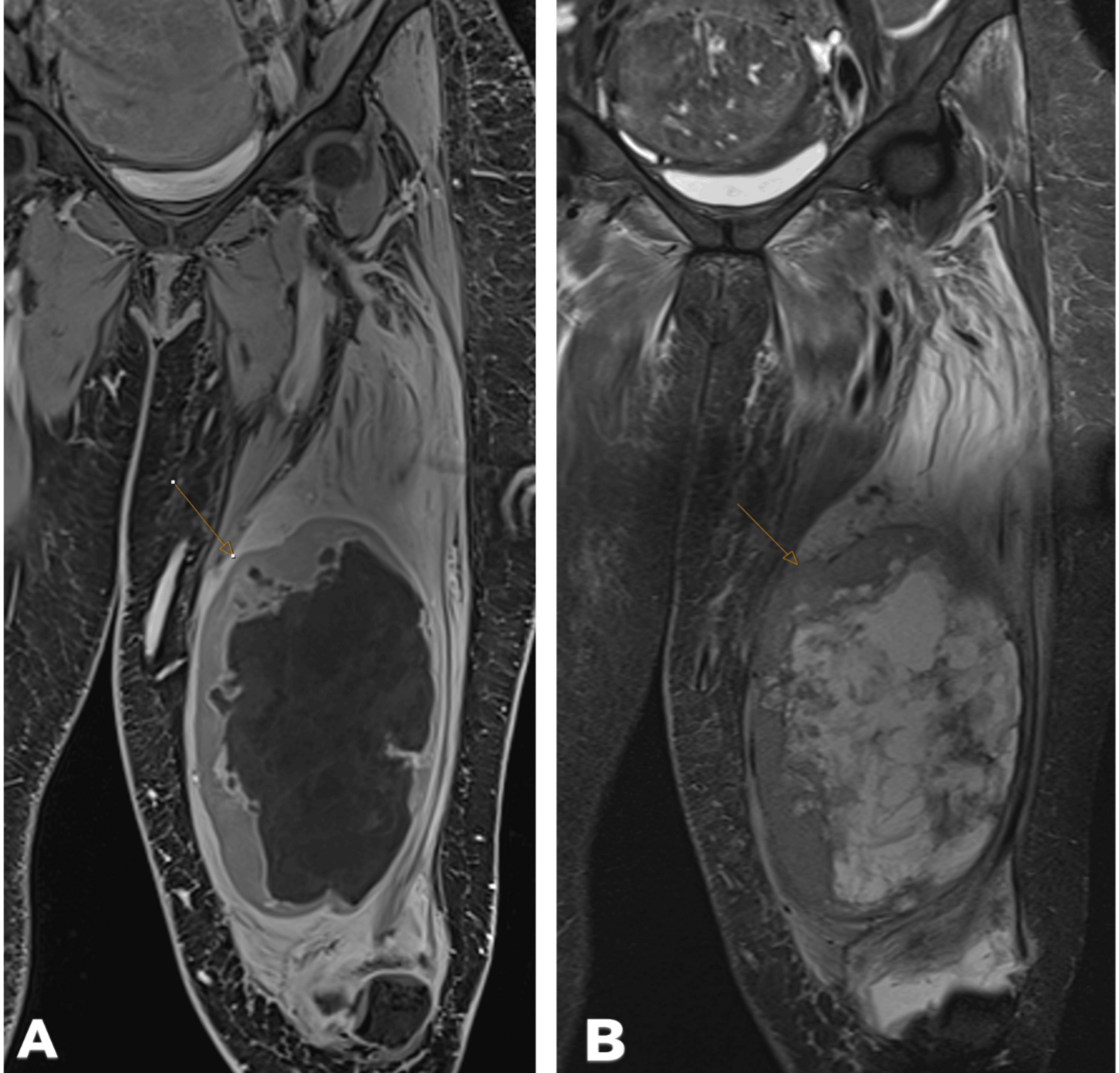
MRI of the mass. A: T2-weighted TSE STIR coronal image. B: Post-contrast T1-weighted VIBE STIR coronal image. The mass measures 225 mm × 78 mm × 74 mm and demonstrates central necrosis (indicated by the arrow) TSE: turbo spin-echo; STIR: short Tau inversion recovery; VIBE: volumetric interpolated breath-hold examination; MRI: magnetic resonance imaging
